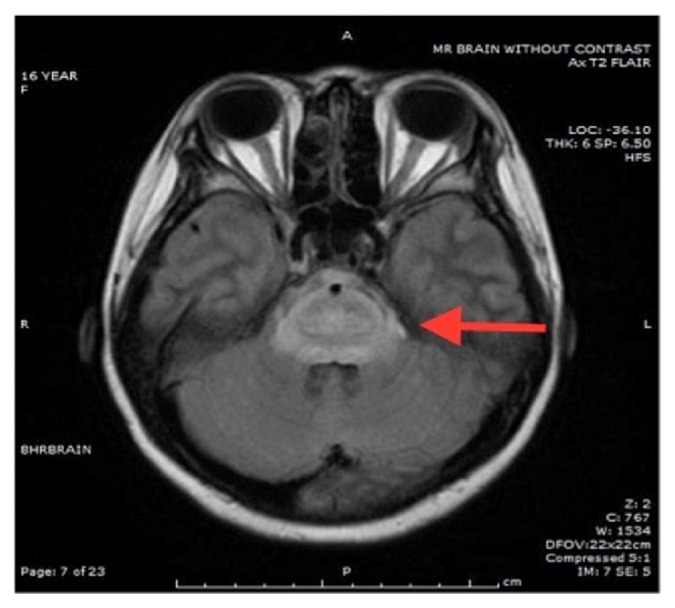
T2-FLAIR Axial image showing diffuse pontine edema.
